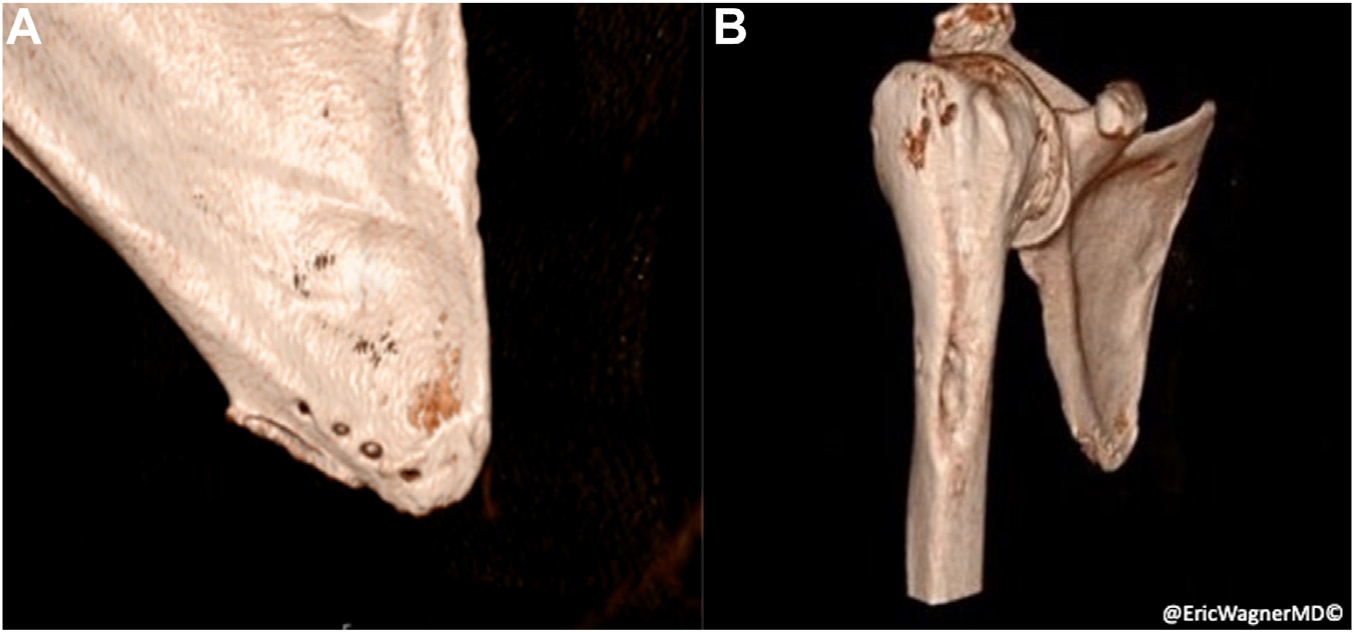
3D reconstructions from 7 months postoperative CT demonstrating osteointegrated humeral bone plug secured with non-absorbable suture looped through transosseous tunnels (A) and donor site at pectoralis major insertion (B). *CT*, computed tomography.
